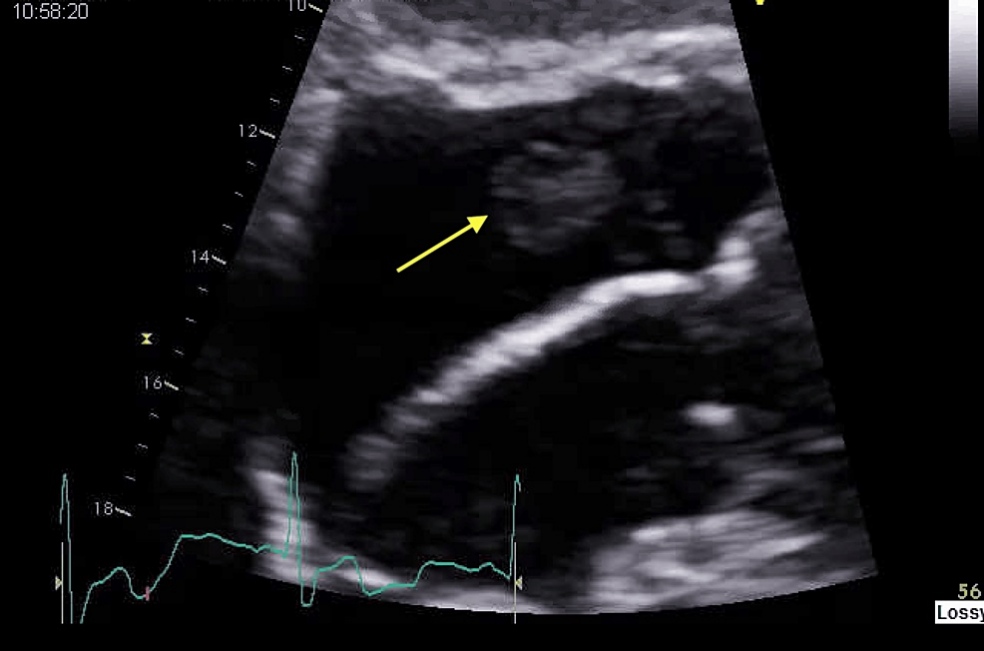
2D echo A large (1.6 x 1.7 cm) round echo dense mass detected on the atrial side of the tricuspid valve is indicated by a yellow arrow in the TTE image. TTE: Transthoracic echocardiogram; 2D: Two-dimensional
